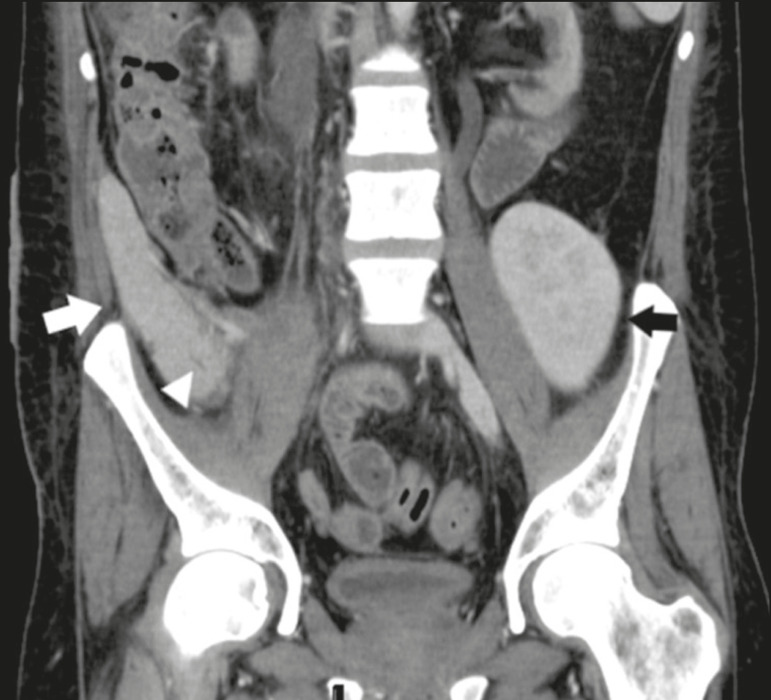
Coronal reconstruction of contrast-enhanced CT, showing normal parenchymal enhancement of the body and tail of the pancreatic graft (white arrow) in the right iliac fossa. A nondilated segment of the pancreatic duct is displayed (arrowhead). Note the renal graft with normal parenchymal enhancement (black arrow) in the left iliac fossa.
